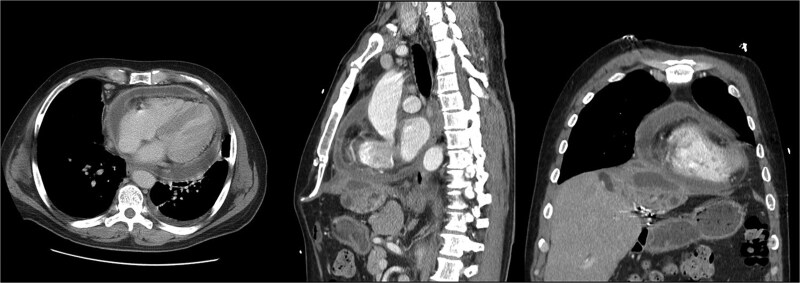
Second readmission imaging—CT of the chest in the axial, sagittal, and coronal views. These demonstrate that the abscess has eroded through the diaphragm and now abuts the pericardium. Additionally, it shows a worsening of the pericardial effusion, concerning for abscess communication.
